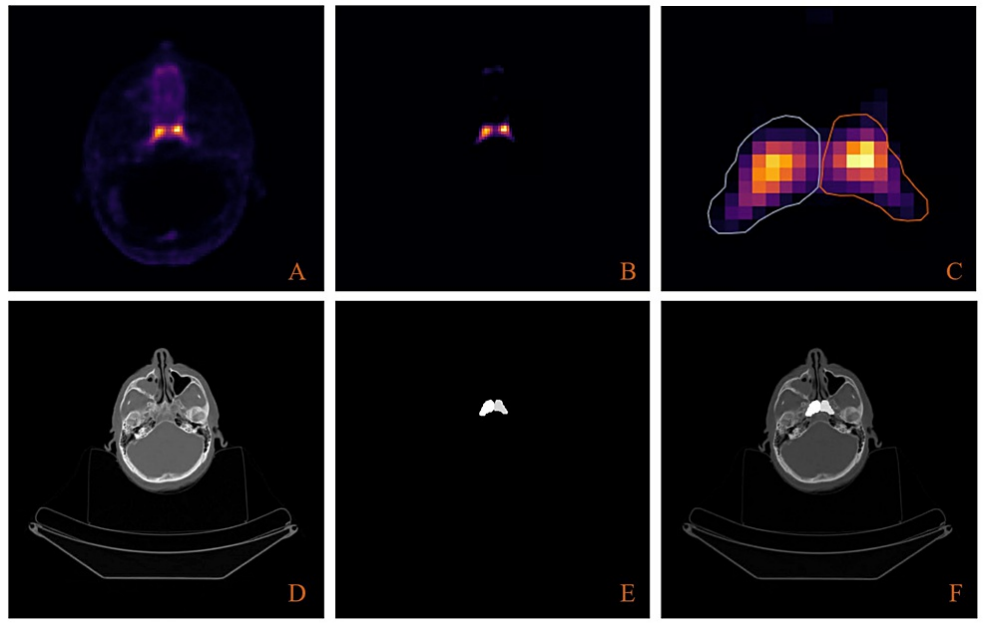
Overview of the manual tubarial gland delineation process. (A) PSMA PET images are converted to SUVbw. (B) A threshold is applied to images to illuminate tubarial gland perimeters. (C) Tubarial glands are contoured according to the threshold-defined perimeter. (D) CT and PET images are registered so that PET-derived contours and CT images use the same coordinate system. (E) Binary masks for each CT slice are created (1 inside the gland, 0 outside). (F) For visualization, the tubarial gland masks are shown overlayed with their corresponding location on the CT image (not used for training). PSMA: prostate-specific membrane antigen; PET: positron emission tomography; SUVbw: standard uptake value based on body weight.
